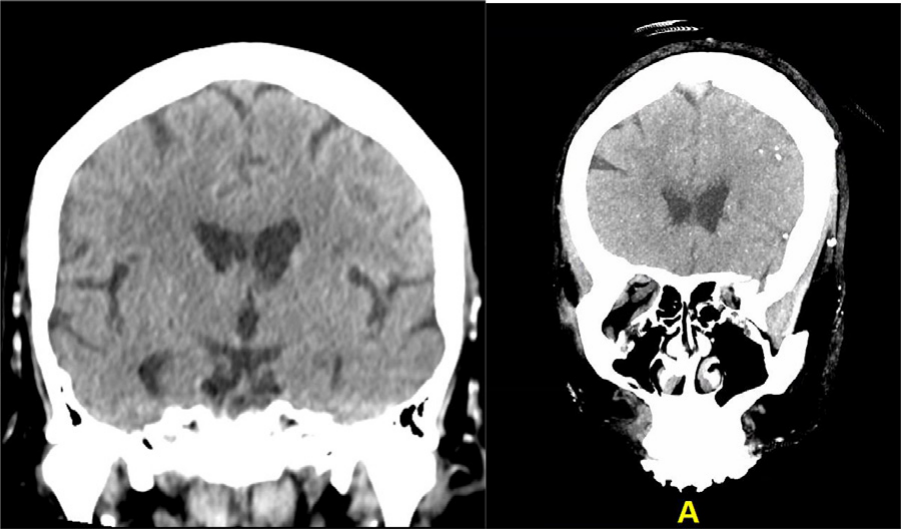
Initial unremarkable coronal CT head without contrast (left); coronal MIP reconstruction of follow up CT head without contrast performed 13 h later showing numerous, new calcified emboli on a single MIP image (right).
